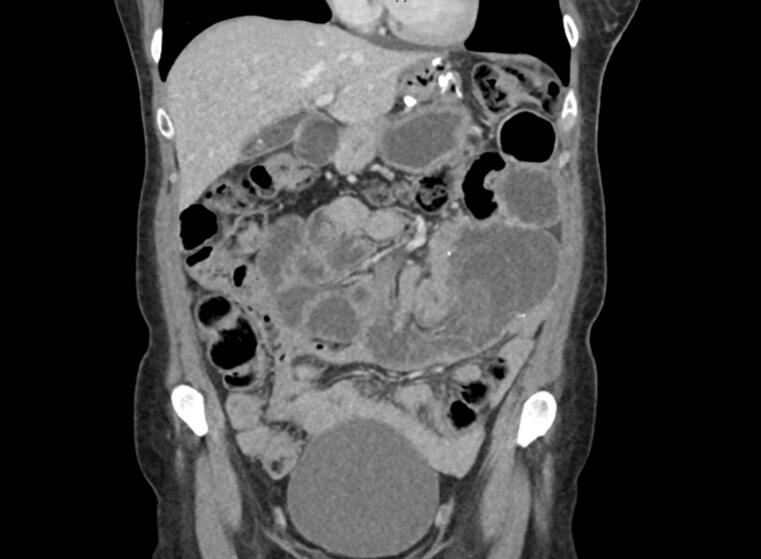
Coronal slice of CT abdomen pelvis in the portal venous phase demonstrating high-grade small bowel obstruction with intussusception at the jejunojenunostomy site in the mid-abdomen.
